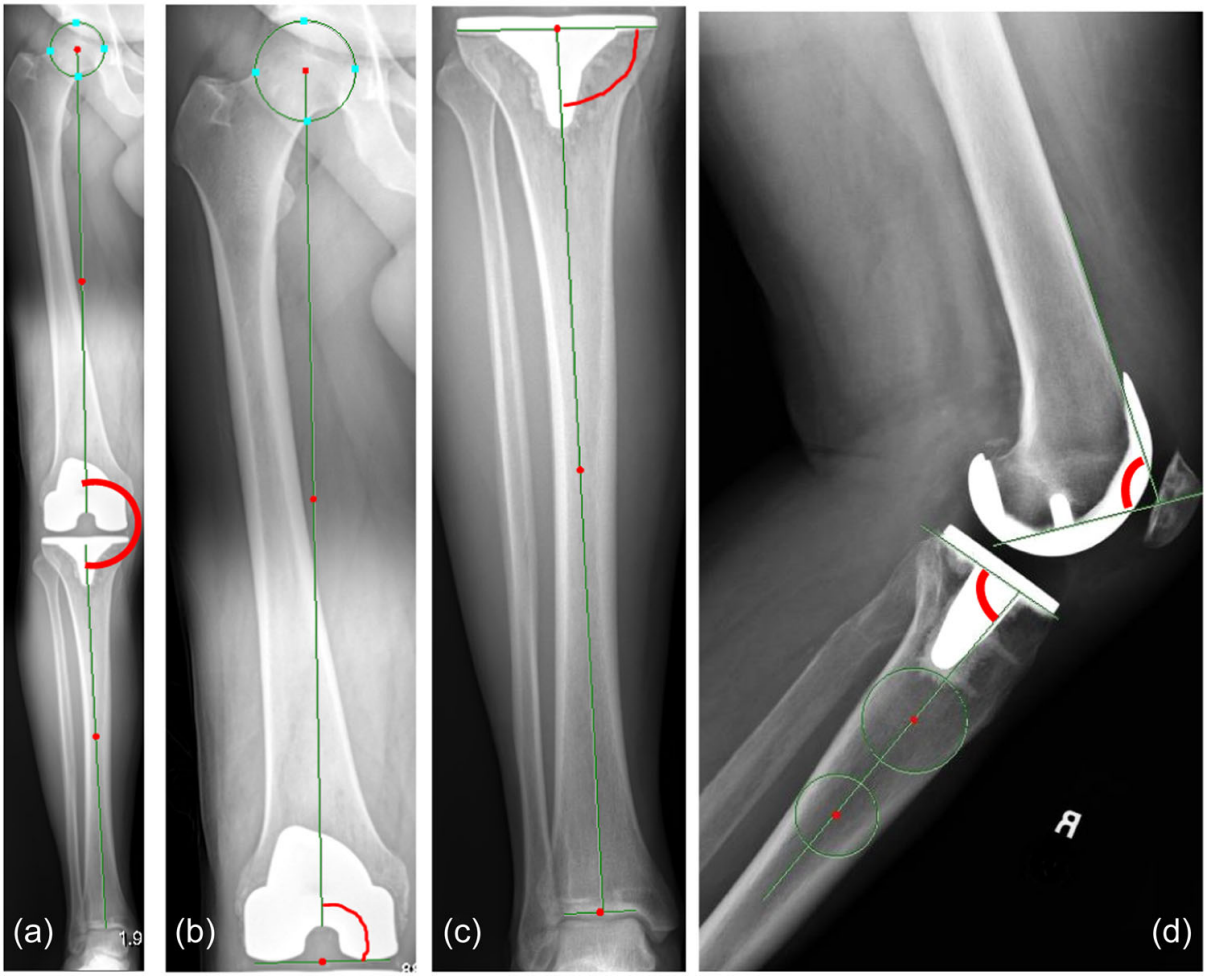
Example x‐ray images with definition of measurements made. (a) Hip–knee–angle measured by taking the angle between the mechanical axis of the femur and the mechanical axis of the tibia. (B) Mechanical medial distal femoral angle. (C) Mechanical medial proximal tibial angle. (D) Tibial posterior slope and femoral flexion. The femoral flexion and posterior tibial slope were measured on a standard view lateral radiograph, this did not allow direct measurement of the angle relative to the mechanical axis.
